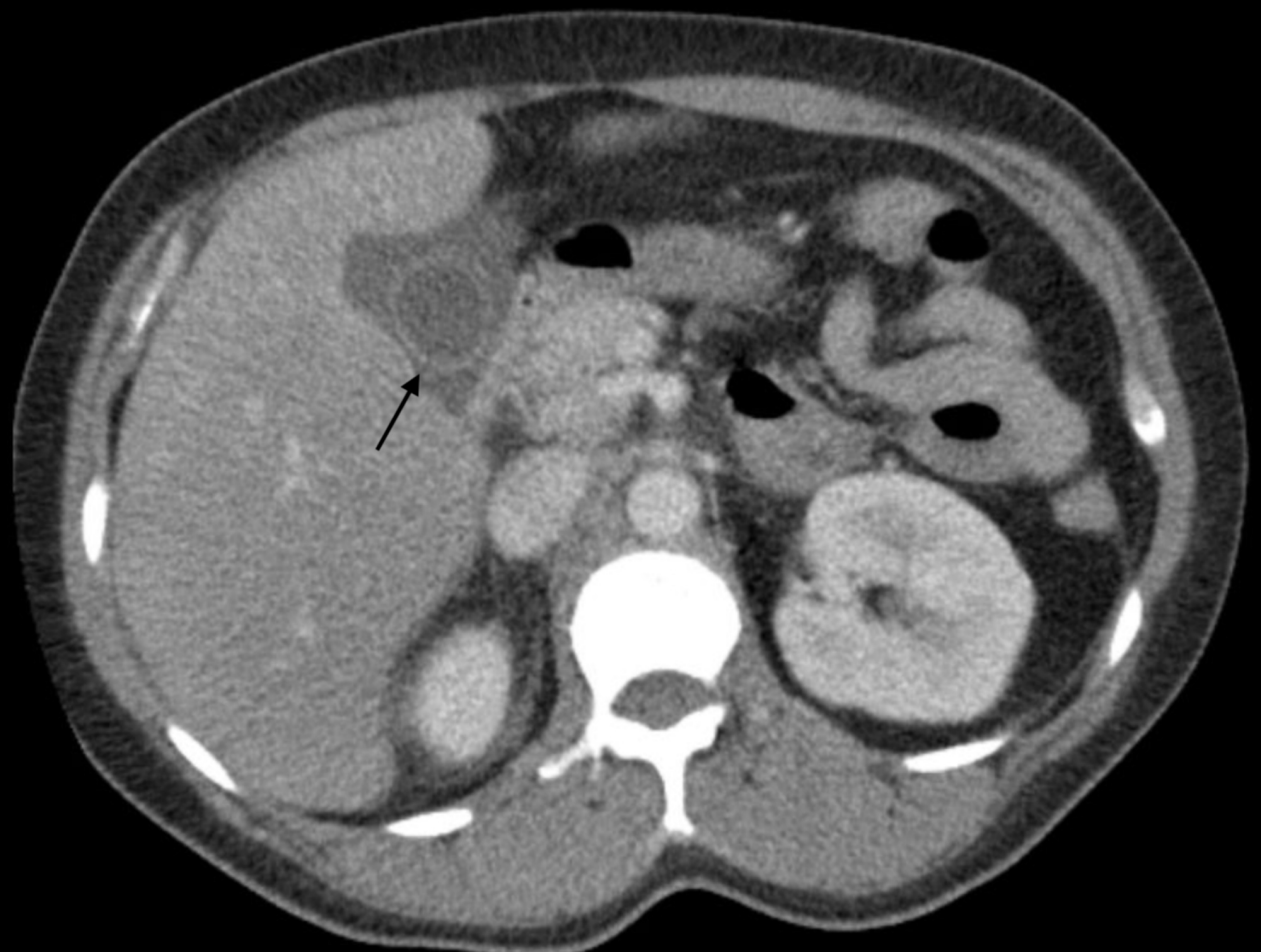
CT Abdomen & Pelvis with Contrast - Before Laparoscopic Cholecystectomy Black arrow: Gallbladder with pericholecystic fluid
